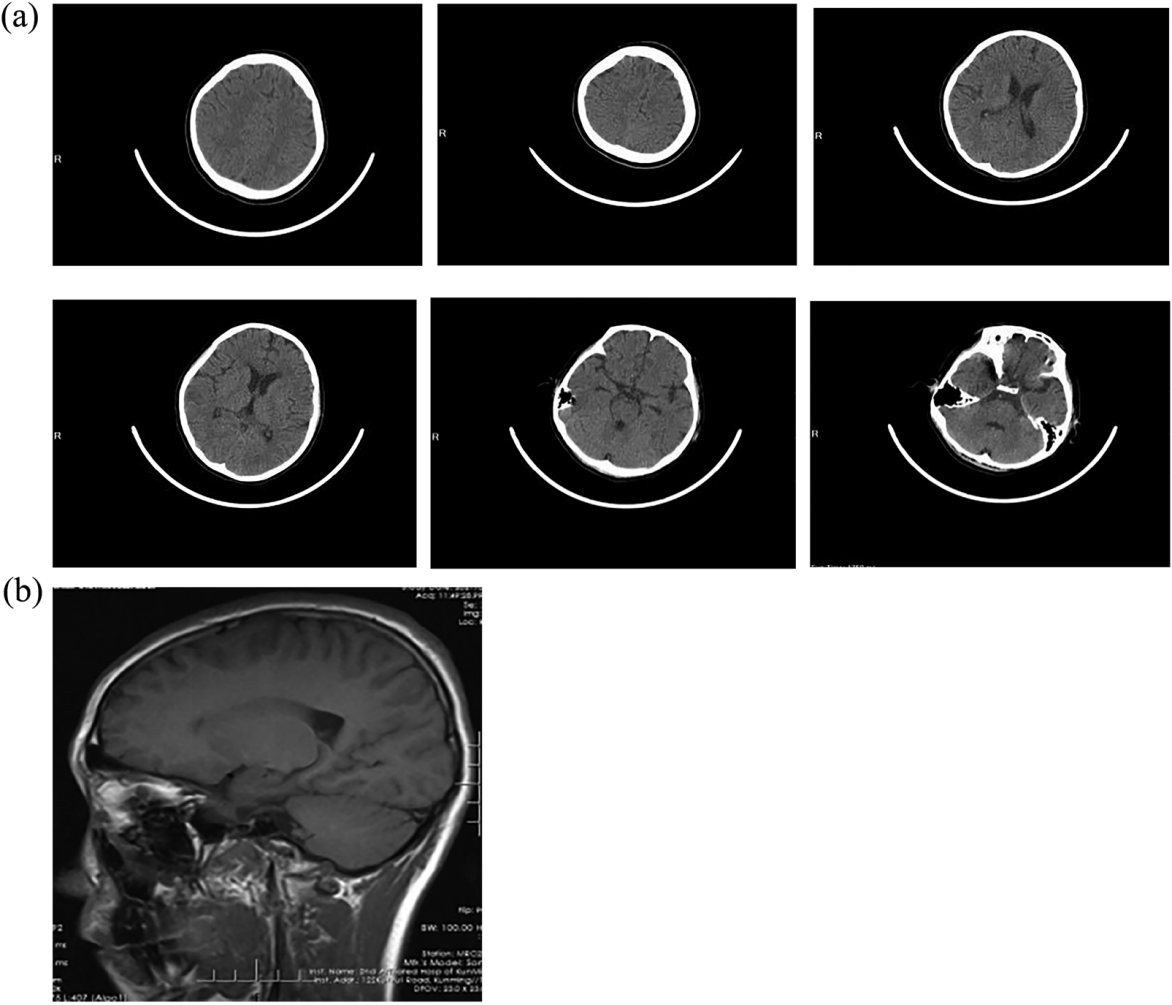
(a) CT image and (b) MRI image.
